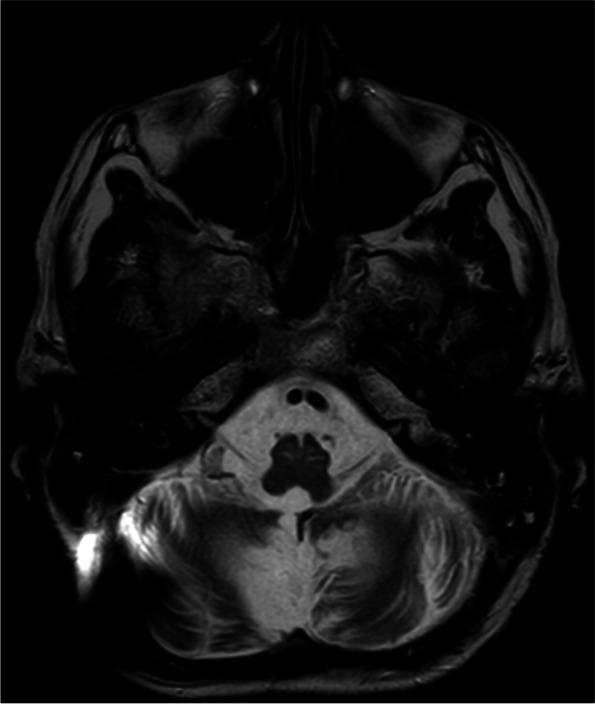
T2-weighted MRI demonstrating hyperintensities within the bilateral inferior olivary nuclei. The absence of inferior olivary nuclei hypertrophy is consistent with stage 3 radiological HOD [1]. Additional findings include cerebellar gliosis and atrophy secondary to previous posterior fossa surgery and radiotherapy
